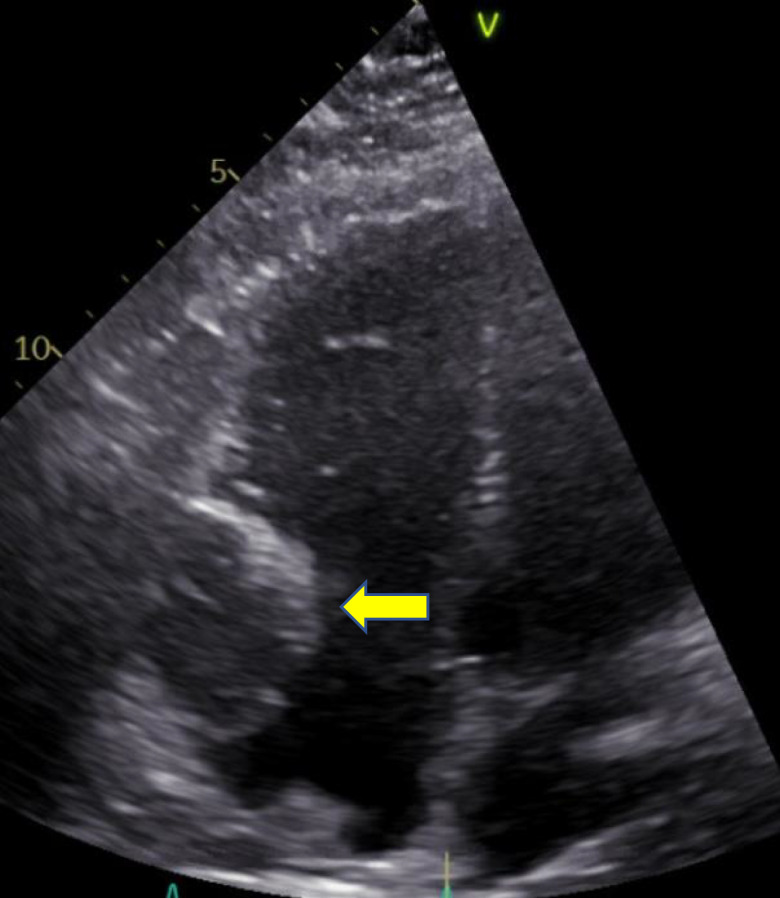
Echocardiogram: large heterogenous mass in the right atrium appearing adherent to the lateral wall.
